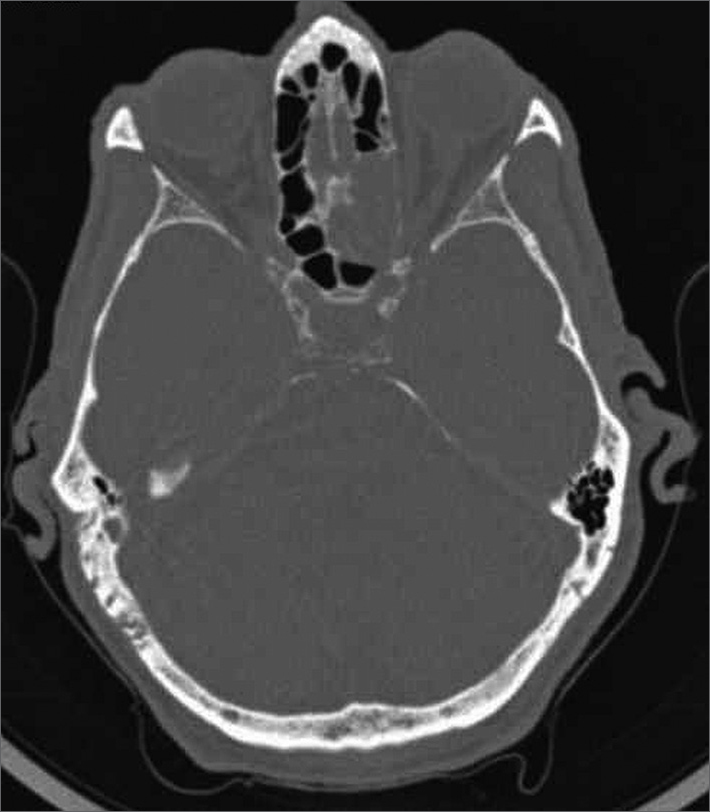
Axial view CT scan: soft-tissue density tumor in left posterior ethmoidal cells measuring 19 × 20 × 30 mm.
